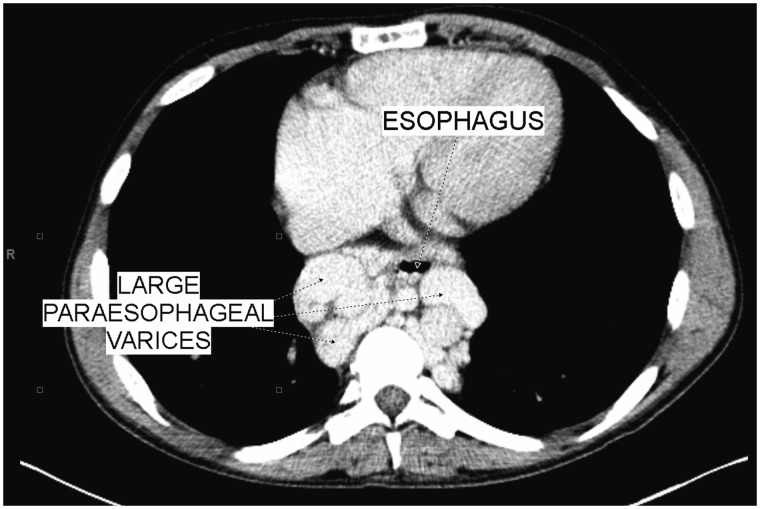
CT scan of the abdomen showing large paraesophageal varices. In this patient, portal vein diameter was measured at 8 mm.
